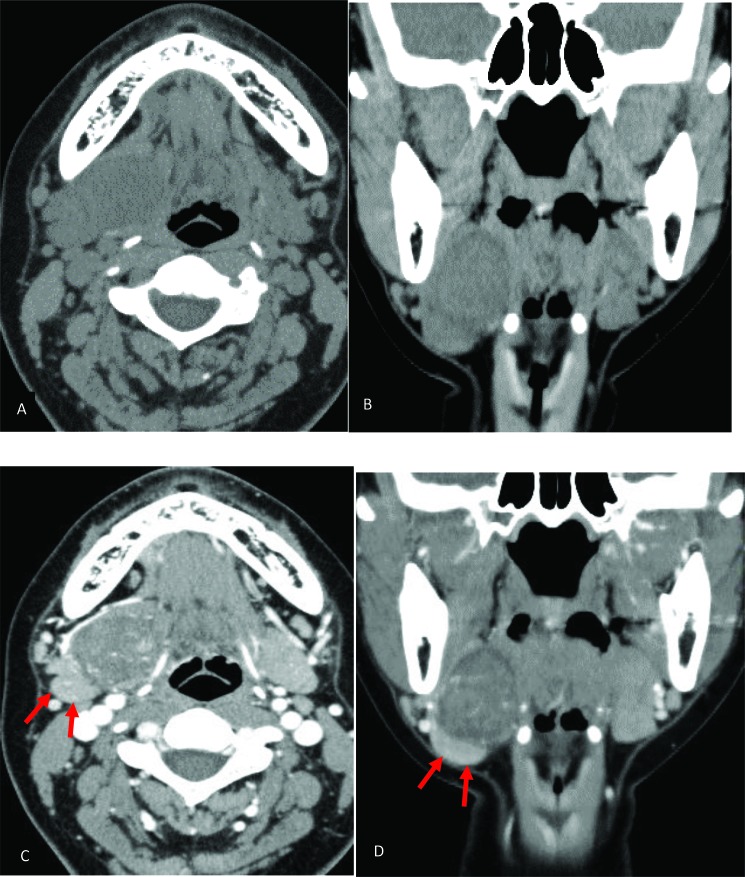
Non-contrast (A, B) and contrast-enhanced (C, D) CT images. The mass was located lateral to the genioglossus and hypoglossus muscles and the submandibular gland was compressed and inferiorly displaced (arrow). Contrast-enhanced CT demonstrated enhancement of intratumoral blood vessels.
